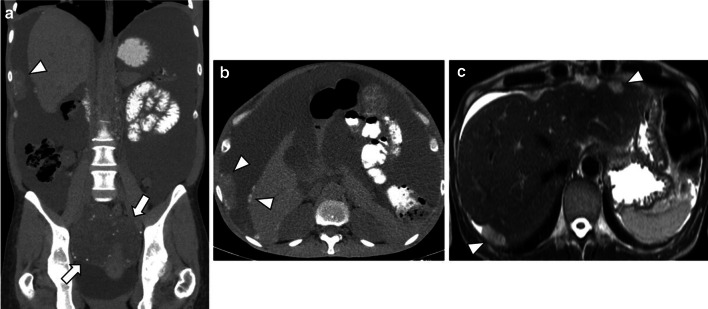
Bilateral LGSC with foci of SBT with peritoneal metastases in a 48-year-old female. Coronal and axial unenhanced CT images (a, b) display a pelvic bilateral cystic tumour (arrows) with several calcified psammoma bodies and calcified peritoneal metastases, namely in the hepatic surface and in the right hypochondrium peritoneum (arrowheads). Severe ascites is also seen, which is a rare manifestation in this type of tumour. Axial T2-weighted MR image (c) demonstrates metastases adherent to the liver surface (arrowheads)
